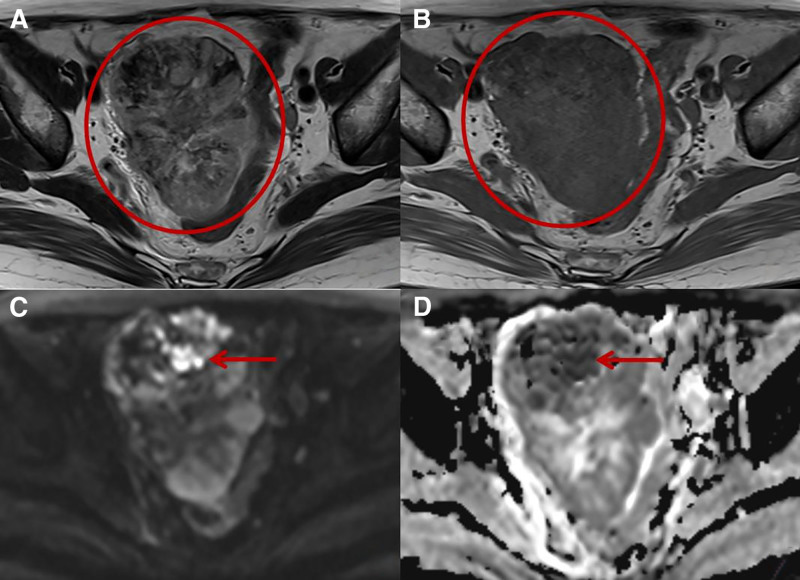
Plain MRI scan of the patient’s pelvic cavity. Axial T2WI (A) and T1WI (B) showed mixed signal solid space-occupying shadows in the right adaccessory area, with a size of 10 × 7 × 7 cm; DWI (C) and ADC (D) (b = 800 s/mm^2^) showed significantly limited local diffusion within the tumor, with an ADC value of approximately 0.447 × 10^−3^ s/mm^2^.
